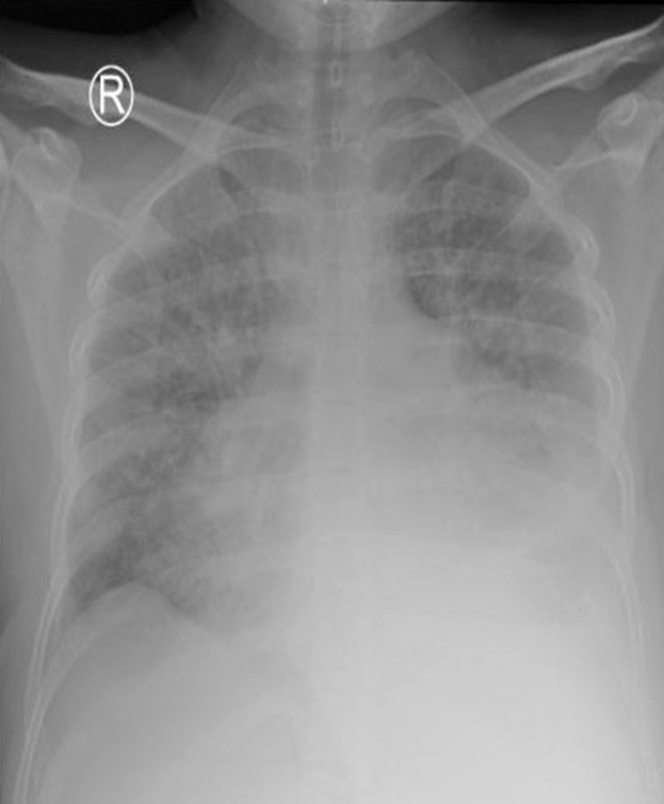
Chest X-Ray at Initial Presentation Chest x-ray at initial presentation showed cardiomegaly with increased pulmonary vascular markings.
